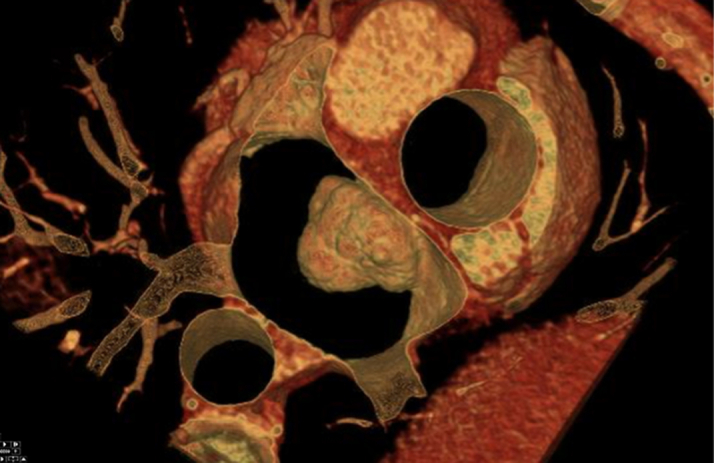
Cardiac Computed Tomography–Derived 3-Dimensional Image Modified surgeons view demonstrating the attachment to the interatrial septum and relationship to the surrounding left atrial anatomy.
